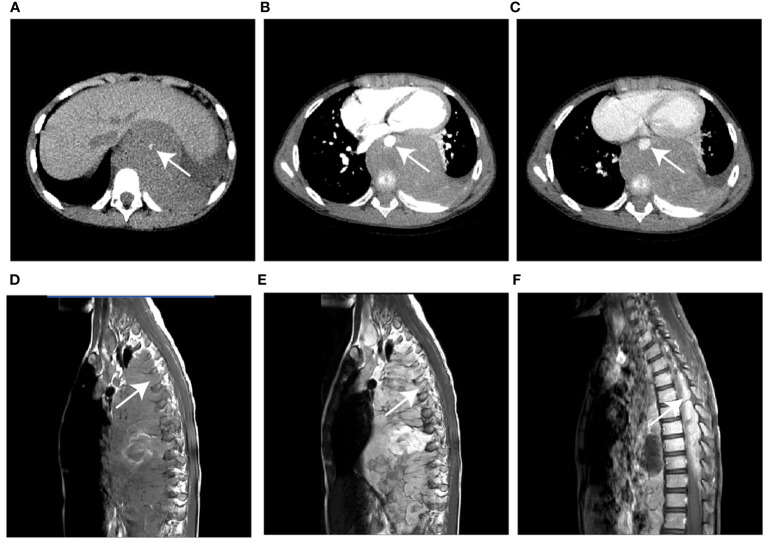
Case 2, a 76-month-old male with NB. (A) The unenhanced CT image shows a hypodense mass in the left posterior mediastinum with punctate calcification (arrow) (B, C) The mass shows heterogeneous enhancement and encases the aorta (arrow) on contrast-enhanced CT images. (D) Sagittal T1WI and (E) T2WI show the soft tissue mass extended into the spinal canal via the intervertebral foramen (arrow). (F) The gadolinium-enhanced T1WI shows the intraspinal extent of the tumor (arrow). WI, weighted image.
